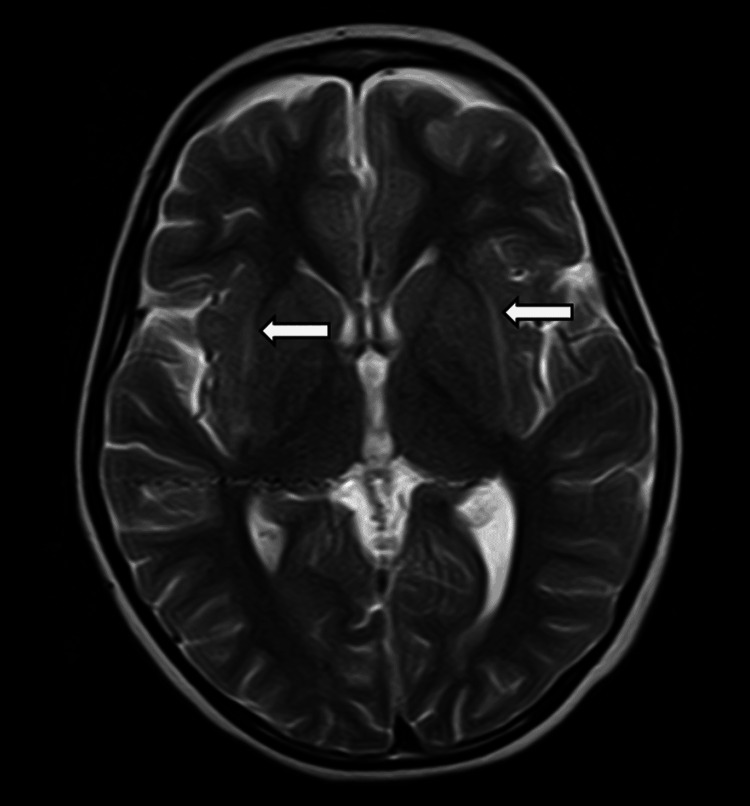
Magnetic resonance imaging of the brain (T2-weighted) White arrows pointing to the hyperintense claustrum.
